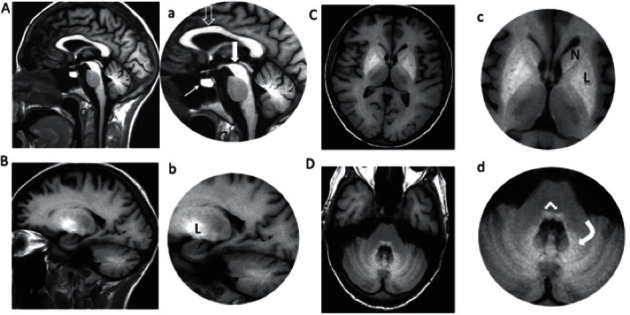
Brain MRI in a patient receiving MnDPDP 140 *μ*mol/kg over 8 months [22, 90]. MnDPDP (10 *μ*mol/kg) was applied as cytoprotective adjunct to 14 cycles of chemotherapy with oxaliplatin as the primary drug in a patient with cancer of colon. MRI of the brain (1.5 T) was undertaken after the last cycle. Sagittal and parasagittal images (A-B, a-b) were obtained by *T*_1_W-FLAIR and descending axial images (C-D, c-d) by *T*_1_W-SE. High SI reflects marked Mn deposition in: A-a, corpus callosum (open arrow), mesencephalon (thick white arrow), and pituitary gland (thin white arrow); B-b, C-c, putamen and globus pallidus (L nucleus lentiformis) and caput nucleus caudatus (N); D-d, cerebellum with nucleus dentatus (curved white arrow) and brain stem (white angled arrow) (Blomlie V, Jynge P., unpublished images).
